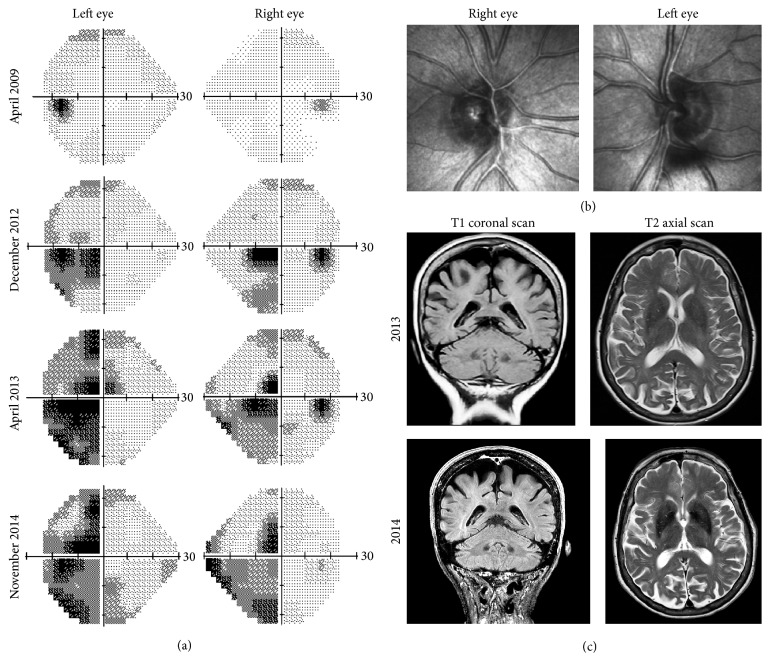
(a) Serial Humphrey visual fields from April 2009 to November 2014 showing progression of superior arcuate defect in the left eye to left-sided incongruous homonymous hemianopia. (b) Optic nerve head imaging in 2014 using the Heidelberg retinal tomography showing no significant disc cupping. (c) Coronal and axial magnetic resonance imaging (without contrast) in 2013 and 2014 showed progressive parietal and occipital cortical atrophy. Posterior cortical atrophy was suspected only in the 2014 scan.
